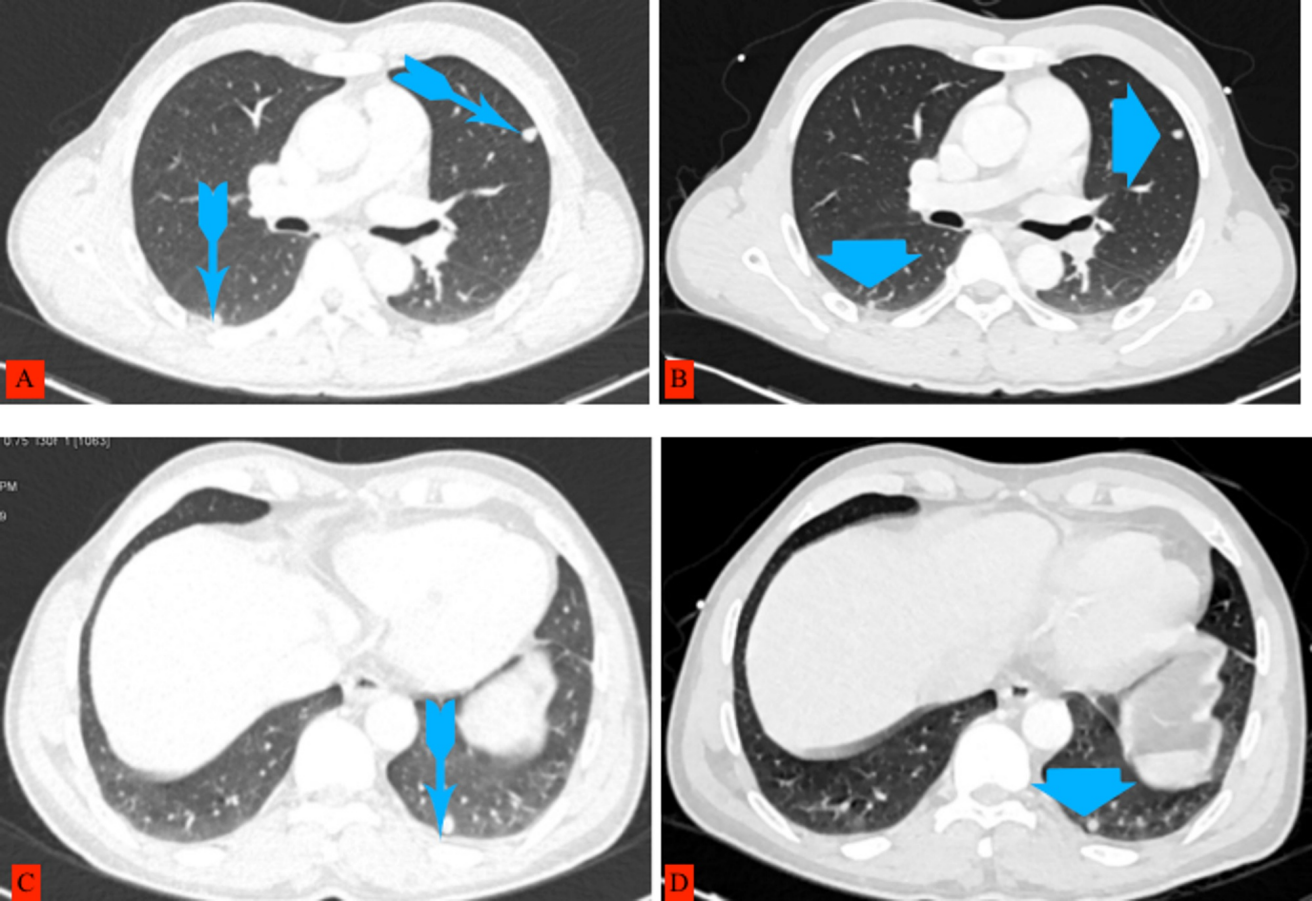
Chest CT images on the left side (A, C) showing nodular lesions pointed with arrows with tails before treatment, and on the right side (B, D), the same lesions pointed with arrowheads but reduced in size six weeks after treatment.
